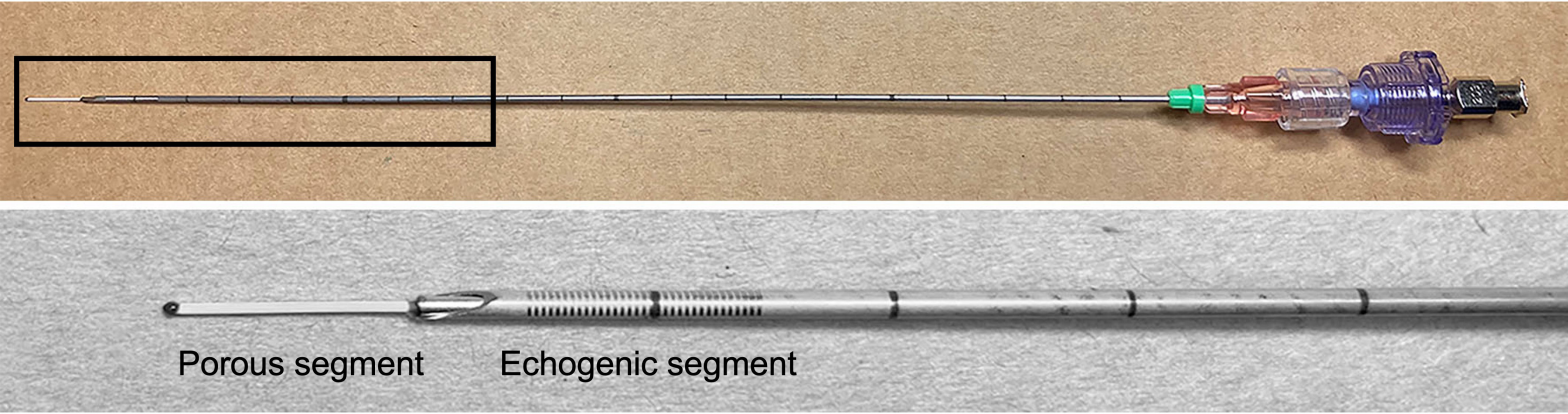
The microporous needle comprises a 20 Gauge cannula body with distal 1 cm porous segment. Included with the cannula is a stabilizing sheath for introducing the microporous segment into tissue target. Sheath has an echogenic sharp tip, which allows for accurate deployment of the microporous segment into the targeted tissue site under the ultrasound control. Top: Once device is in position, the sheath is retracted exposing the porous segment for injection. Bottom: High power magnification of the distal portion of the needle with the retracted stabilizing sheath.
